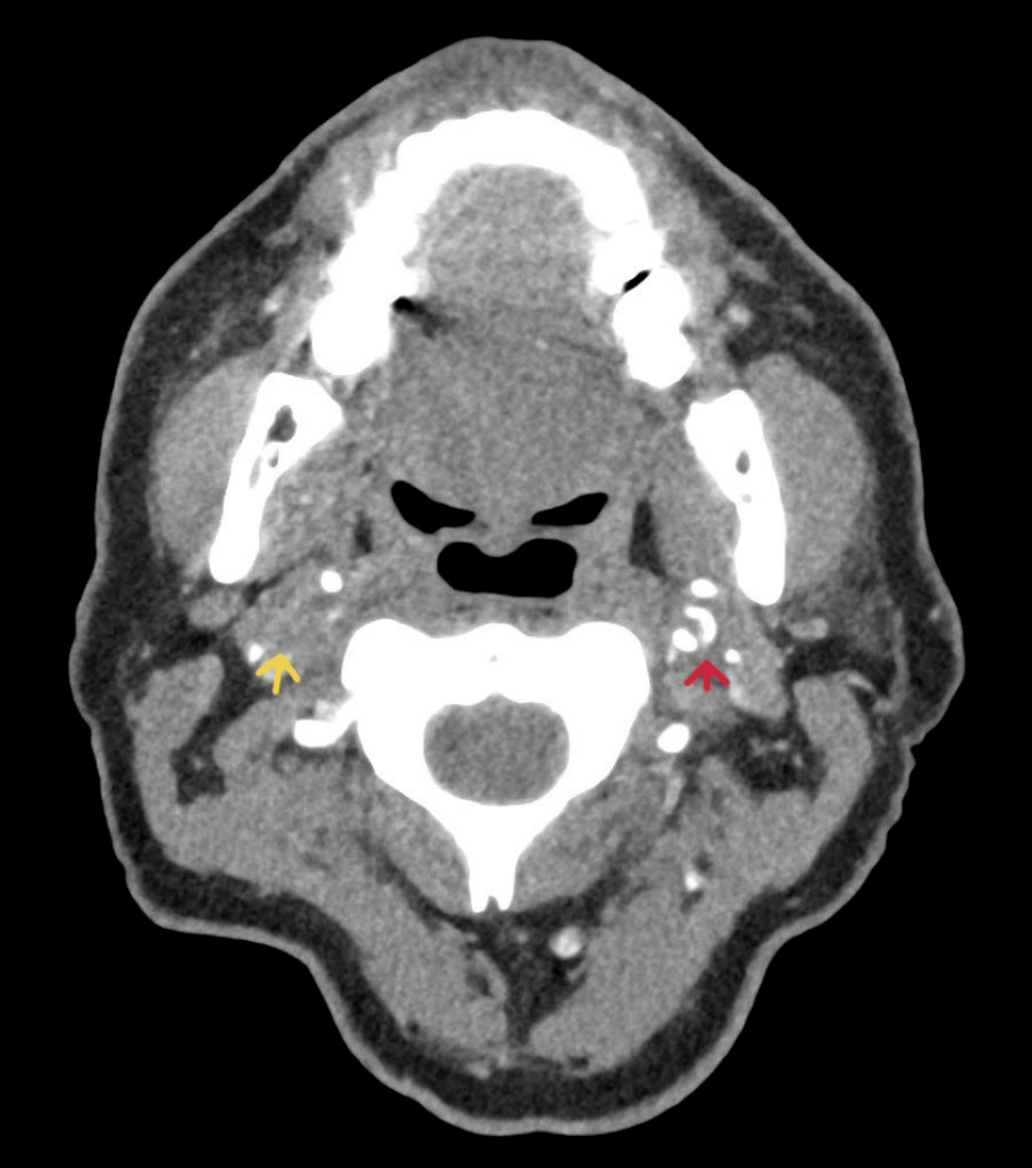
CT angiogram showed a completely occluded right internal carotid artery (yellow arrow), and a severely dissected left internal carotid artery (red arrow) showing true and false lumen.
